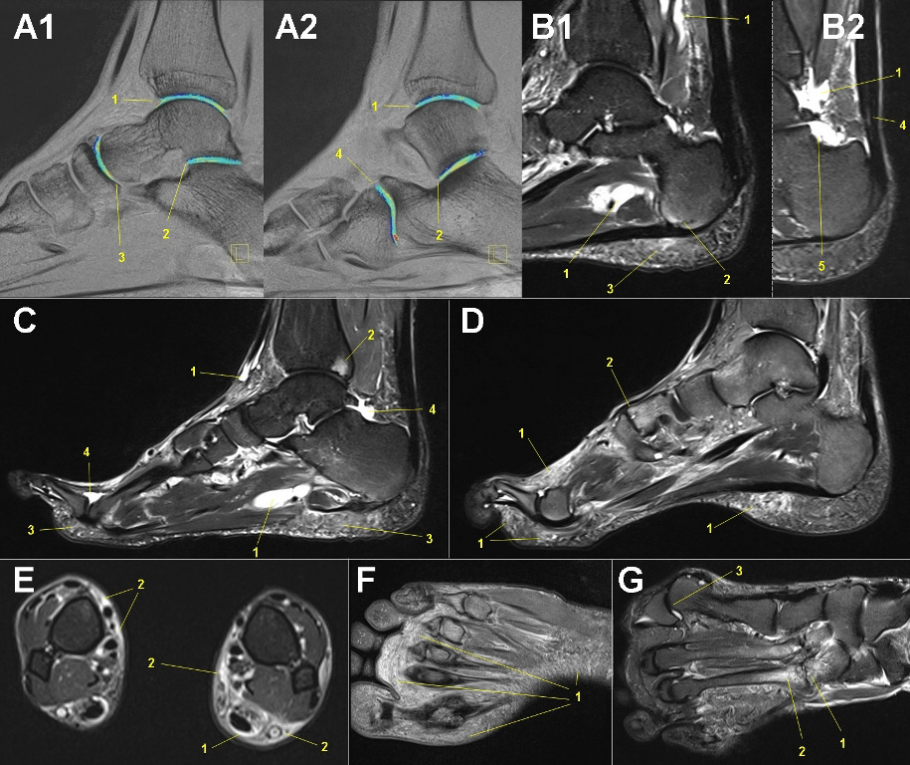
Mobile MRI of the feet. A: male, 39-years-old, stage 53, 3,669 km (fused colored T2* GRE map sagittal: syngo™ MapIt fusion technique, Siemens Medical solutions, Erlangen, Germany): Colored visualization of focal water concentration in cartilage layers of the ankle joint (1), subtalar joint (2), talonavicular joint (3) and calcneocuboid joint (4). B: male, 61-years-old, stage 23, 2,176 km (T2 TIRM sagittal): Multiple running related signs of tissue alteration: Peritendinous fluid accumulation in tendon sheaths (1). Focal bone edema at insertion of plantar fascia (2) with corresponding subcutaneous edema (3), but without plantar fasciitis. Edema in the Achilles tendon (4). Articular effusion in the ankle joint (5). C: male, 59-years-old, stage 45, 3,082 km (T2 TIRM sagittal): Peritendinous fluid accumulation in tendon sheaths of foot dorsiflexors (1), focal arthrosis of the ankle joint with subchondral bone edema (2), plantar subcutaneous edema (3), articular effusion in subtalar and metatarsophalangeal joint (4). D: male, 54-years-old, stage 32, 2,176 km (T2 TIRM sagittal): Subcutaneous edema (1), arthrosis of midfoot joints (2). E: male, 30-years-old, stage 12, 789 km (PDw TSE fs transversal): Peritendinous fluid accumulation in Achilles tendon sheath (1) with wide local subcutaneous edema (2). F: female, 68-years old, stage 15, 1,003 km (PDw TSE fs transversal): Extensive plantar subcutaneous edema (1). G: male, 47-years-old, stage 52, 3,609 km (PDw TSE fs transversal): Severe arthrosis of midfoot joints (1) with perifocal bone edema (2), hallux valgus (3).
